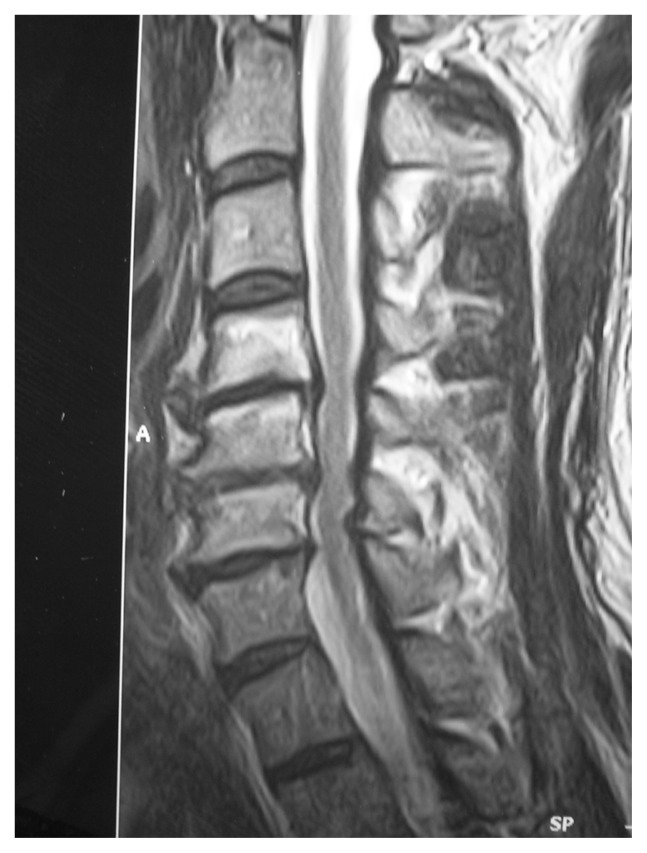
Sagittal T2-w MRI demonstrating degenerative disc protrusions and anterior osteophytes compressing on the esophagus at C4–7 levels.
